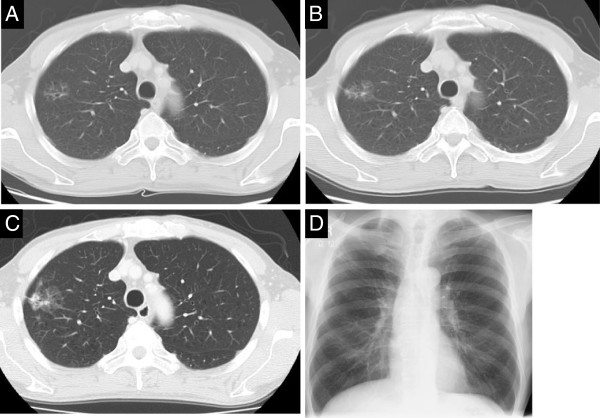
Chest computed tomography and X-ray photography. (A) Computed tomography, June 2009. (B) Computed tomography, January 2011. (C) Computed tomography, March 2012. (D) Chest X-ray photography, March 2012. The GGO at the lateral aspect of right S1 was enlarging, and it became a mass. GGO: ground glass opacity.
